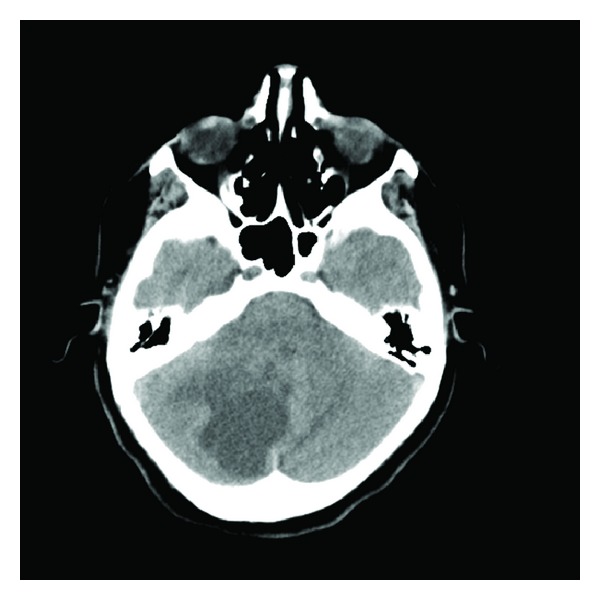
The preop CT scan showed a right cerebellar hypodens lesion.
